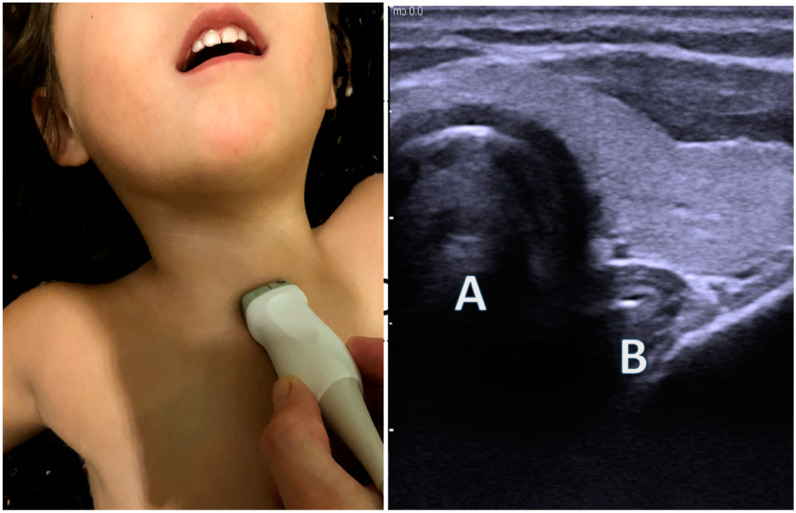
Image showing the placement of a high-frequency linear transducer just above the sternal notch with resultant ultrasound images. A = trachea and B = esophagus.
